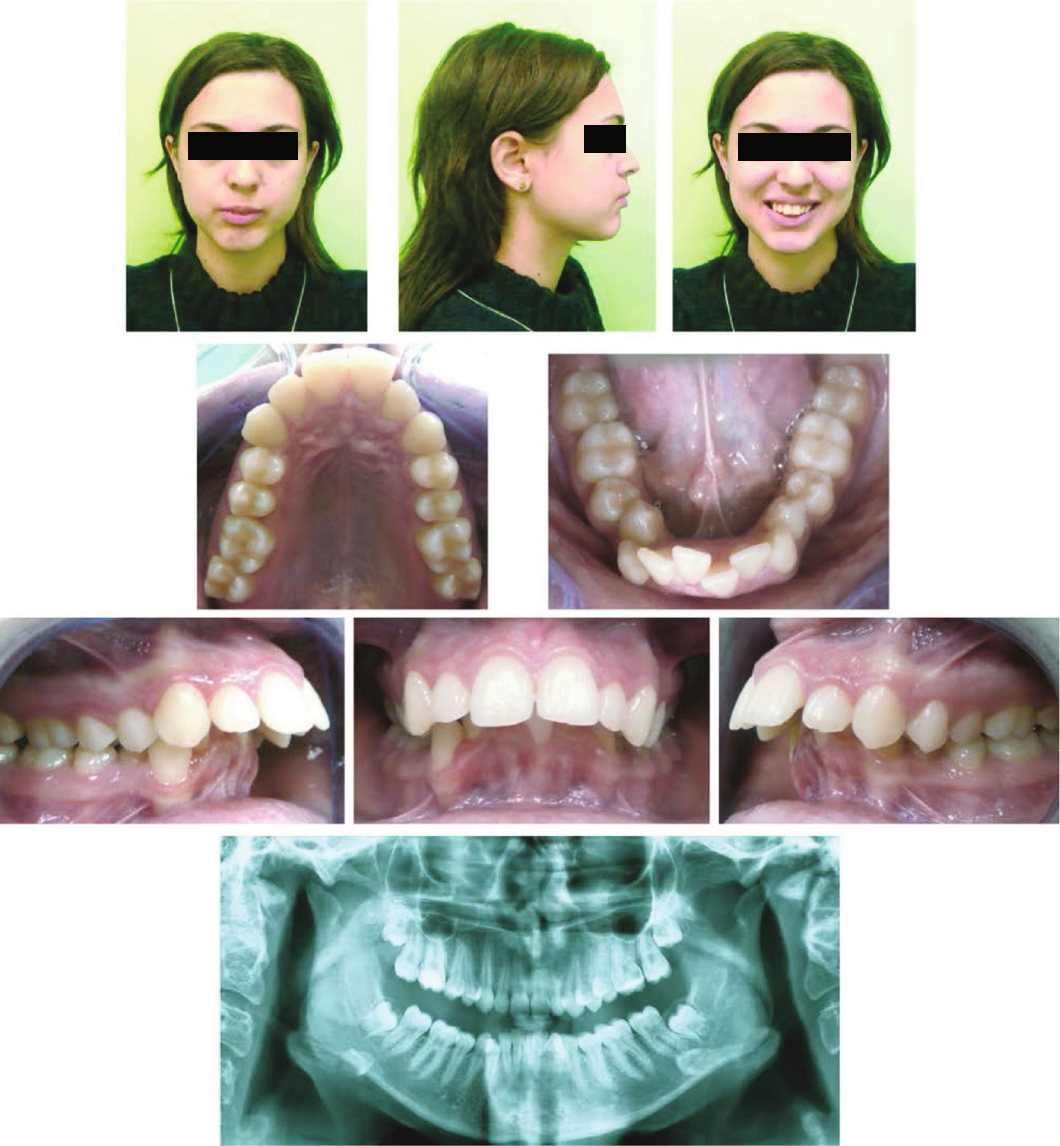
Extraoral photographs (T0), intraoral photographs (T0), and orthopantomogram (T0); T0=pretreatment.
